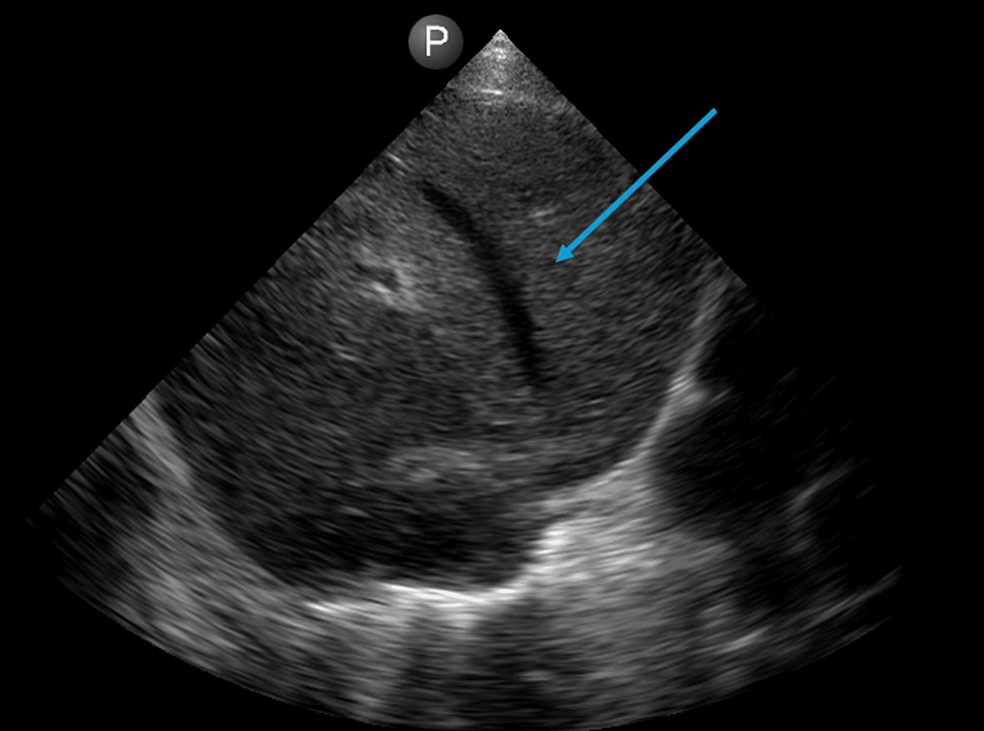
POCUS image at the time of discharge from the clinic demonstrating complete resolution of the abscess. POCUS: point-of-care ultrasound. The blue arrow indicates the liver.
